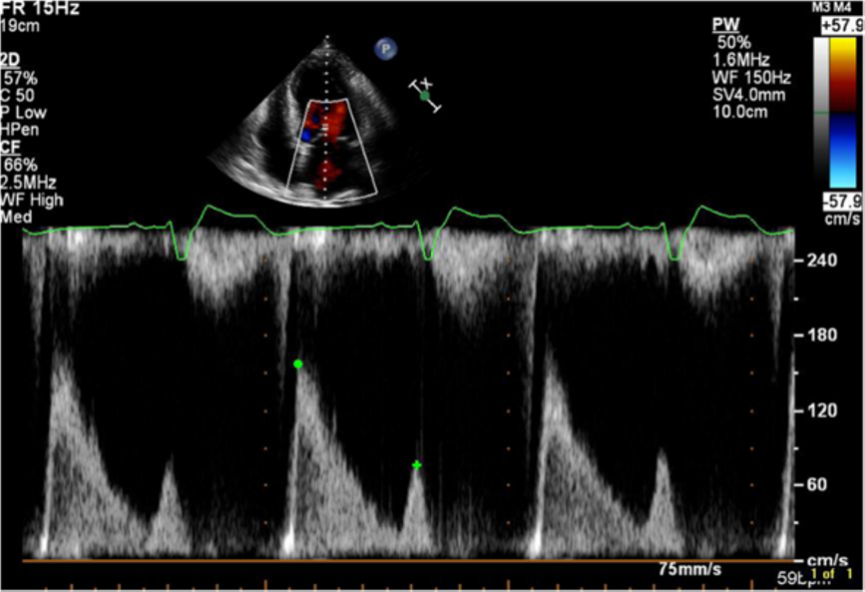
Pulsed wave Doppler of transmitral flow. Pulsed wave Doppler interrogation from the apical four chamber view of the transmitral diastolic flows into the LV can provide adjunct information to grading of MR severity. In this example, the E wave measures approximately 1.6 m/s, and this is consistent with a high flow rate of early diastolic passive LV filling which can be seen with severe MR.
